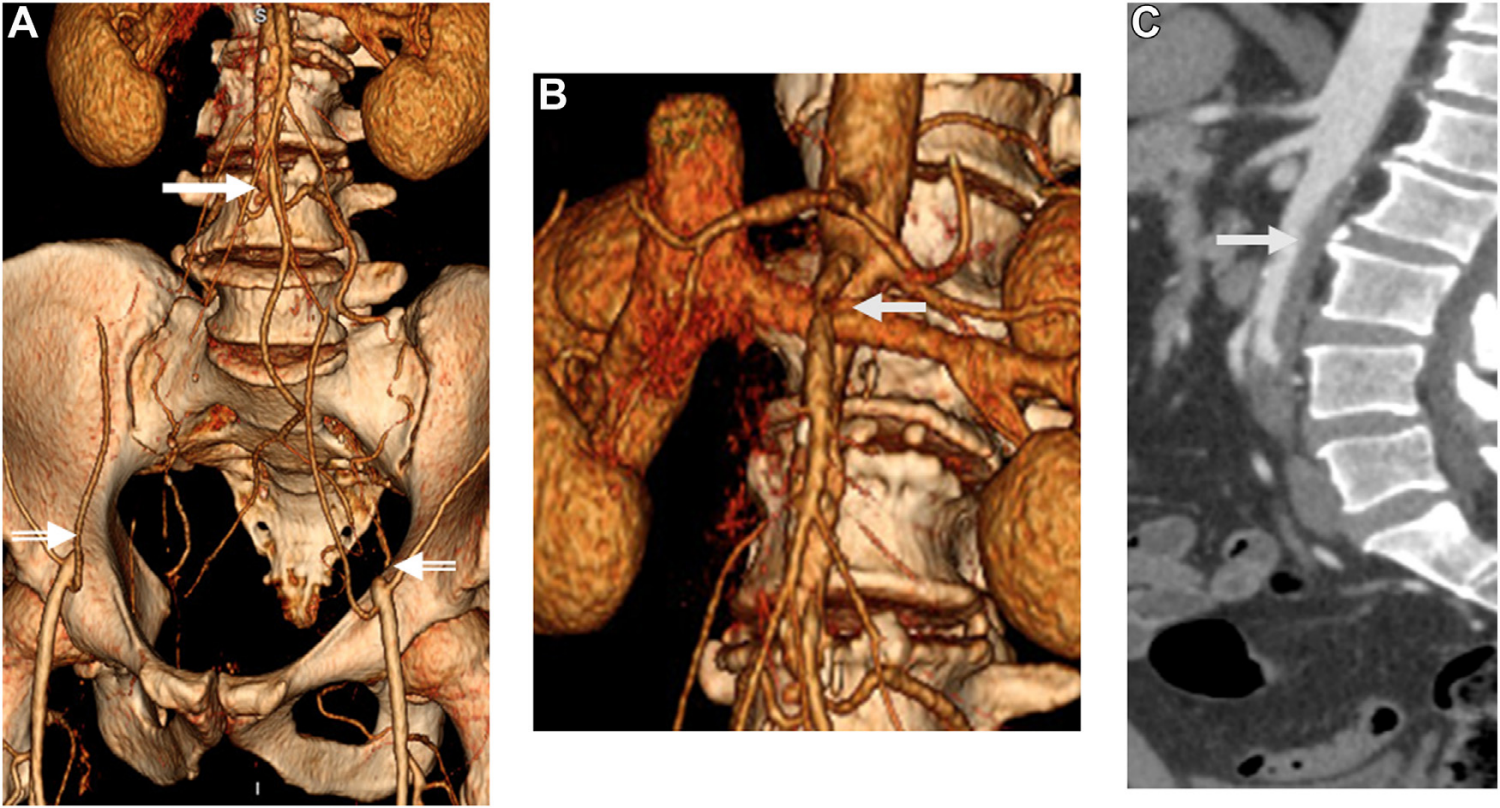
A, Three-dimensional computed tomography reconstruction demonstrating aortic occlusion starting just distal to the takeoff of a large inferior mesenteric artery (IMA; *solid white arrow*). The common, internal, and external iliac arteries were also chronically occluded. The distal external iliac arteries were reconstituted via the inferior epigastric artery, bilaterally (*interrupted white arrows*). B, The superior mesenteric artery (SMA) had severe stenosis at 3 cm from its origin. C, A sagittal computed tomography scan showing extensive, aortic soft plaque starting at the level of the SMA and causing significant stenosis.
